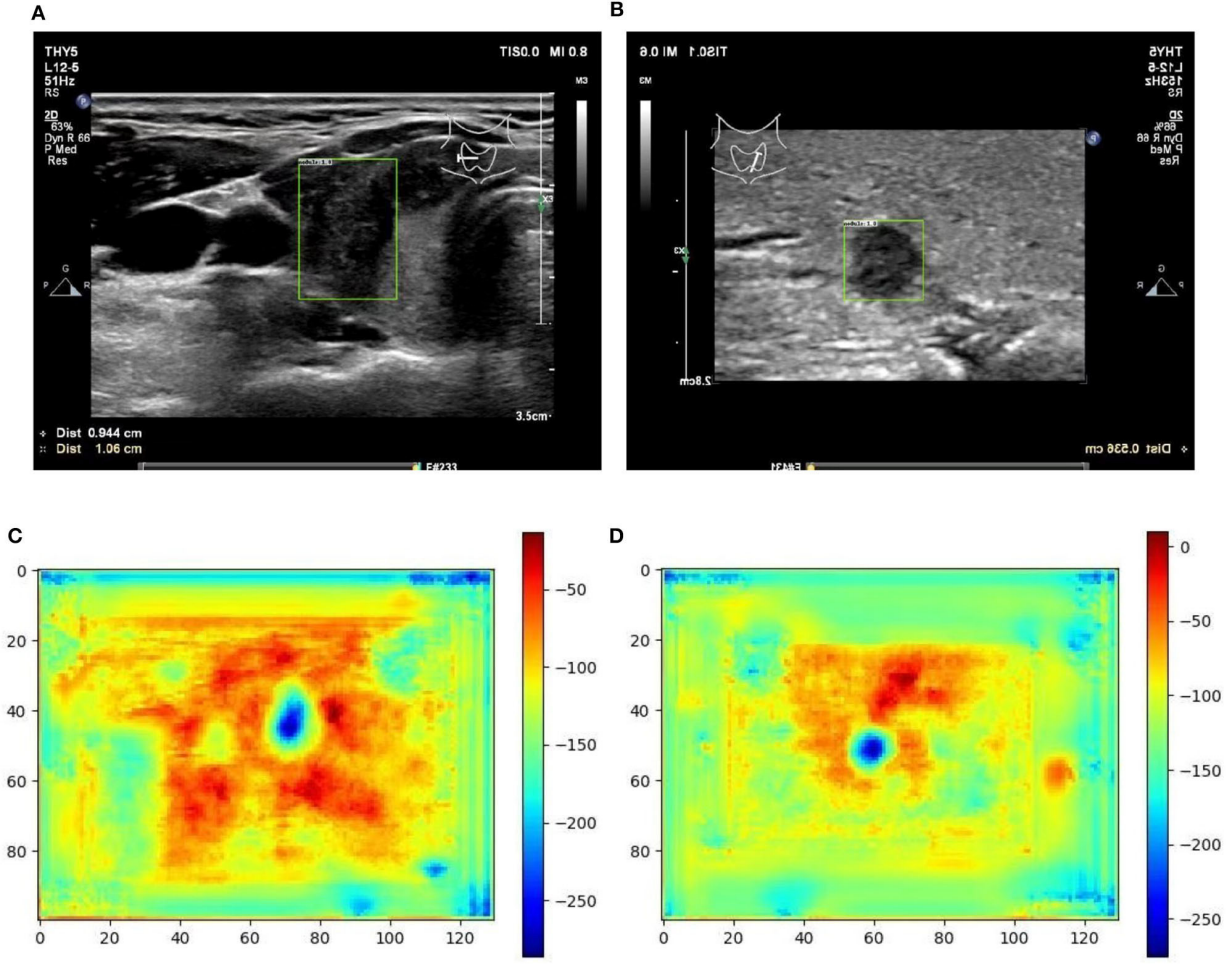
Two examples (from left to right, correspond to the third two-rows in the content of Table 1) of nodule areas which are marker-free as detected by the bounding box in the ultrasound thyroid image, and their corresponding response heat map as visualized by the feature map in the detection part of the networks. Note the heat maps are computed on the model trained by samples whose markers are removed by manual (IP). Best viewed in color. (A) Nodule in ultrasound thyroid image. (B) Nodule in ultrasound thyroid image. (C) Corresponding response heat map. (D) Corresponding response heat map.
